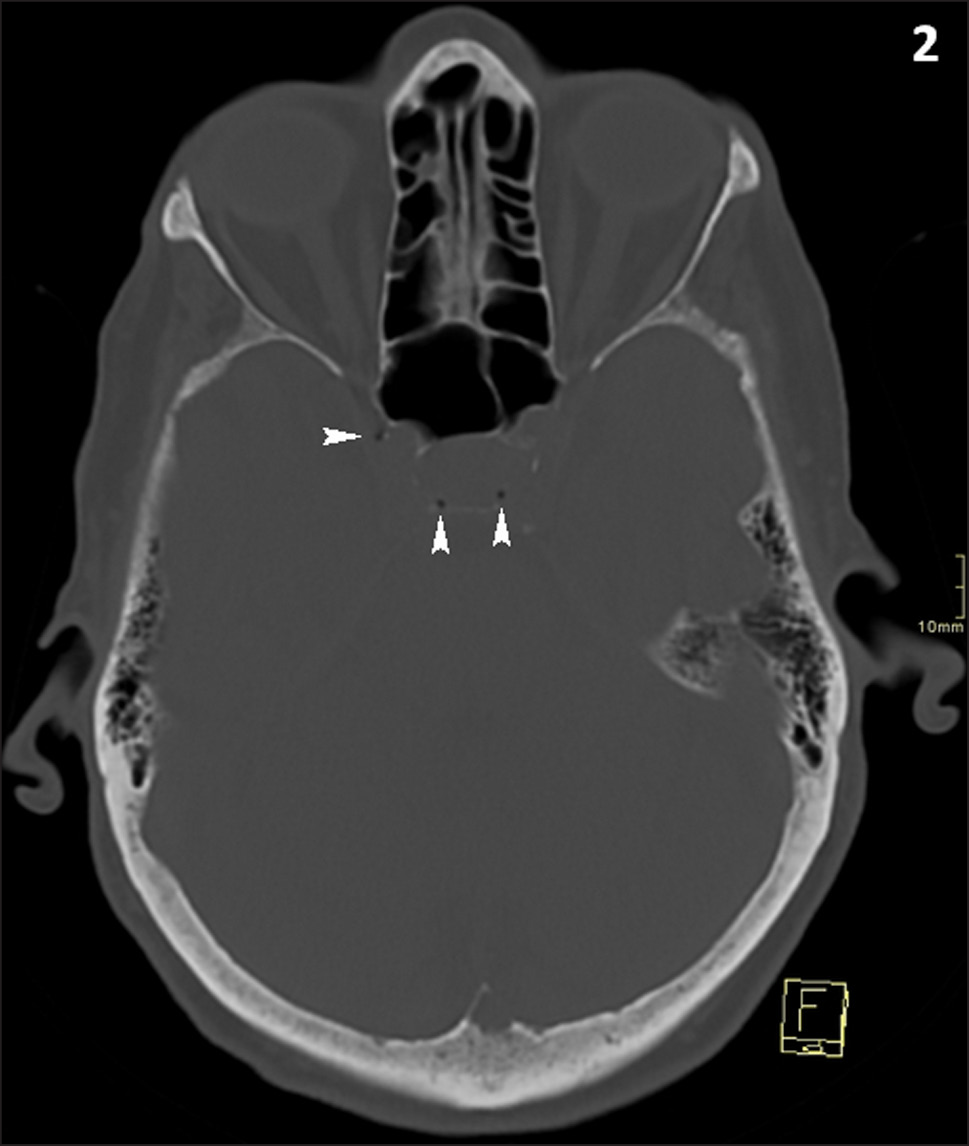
CT of the skull, bone window, axial reformatted image. Air bubbles at sinus intercavernosus (white arrowheads).
